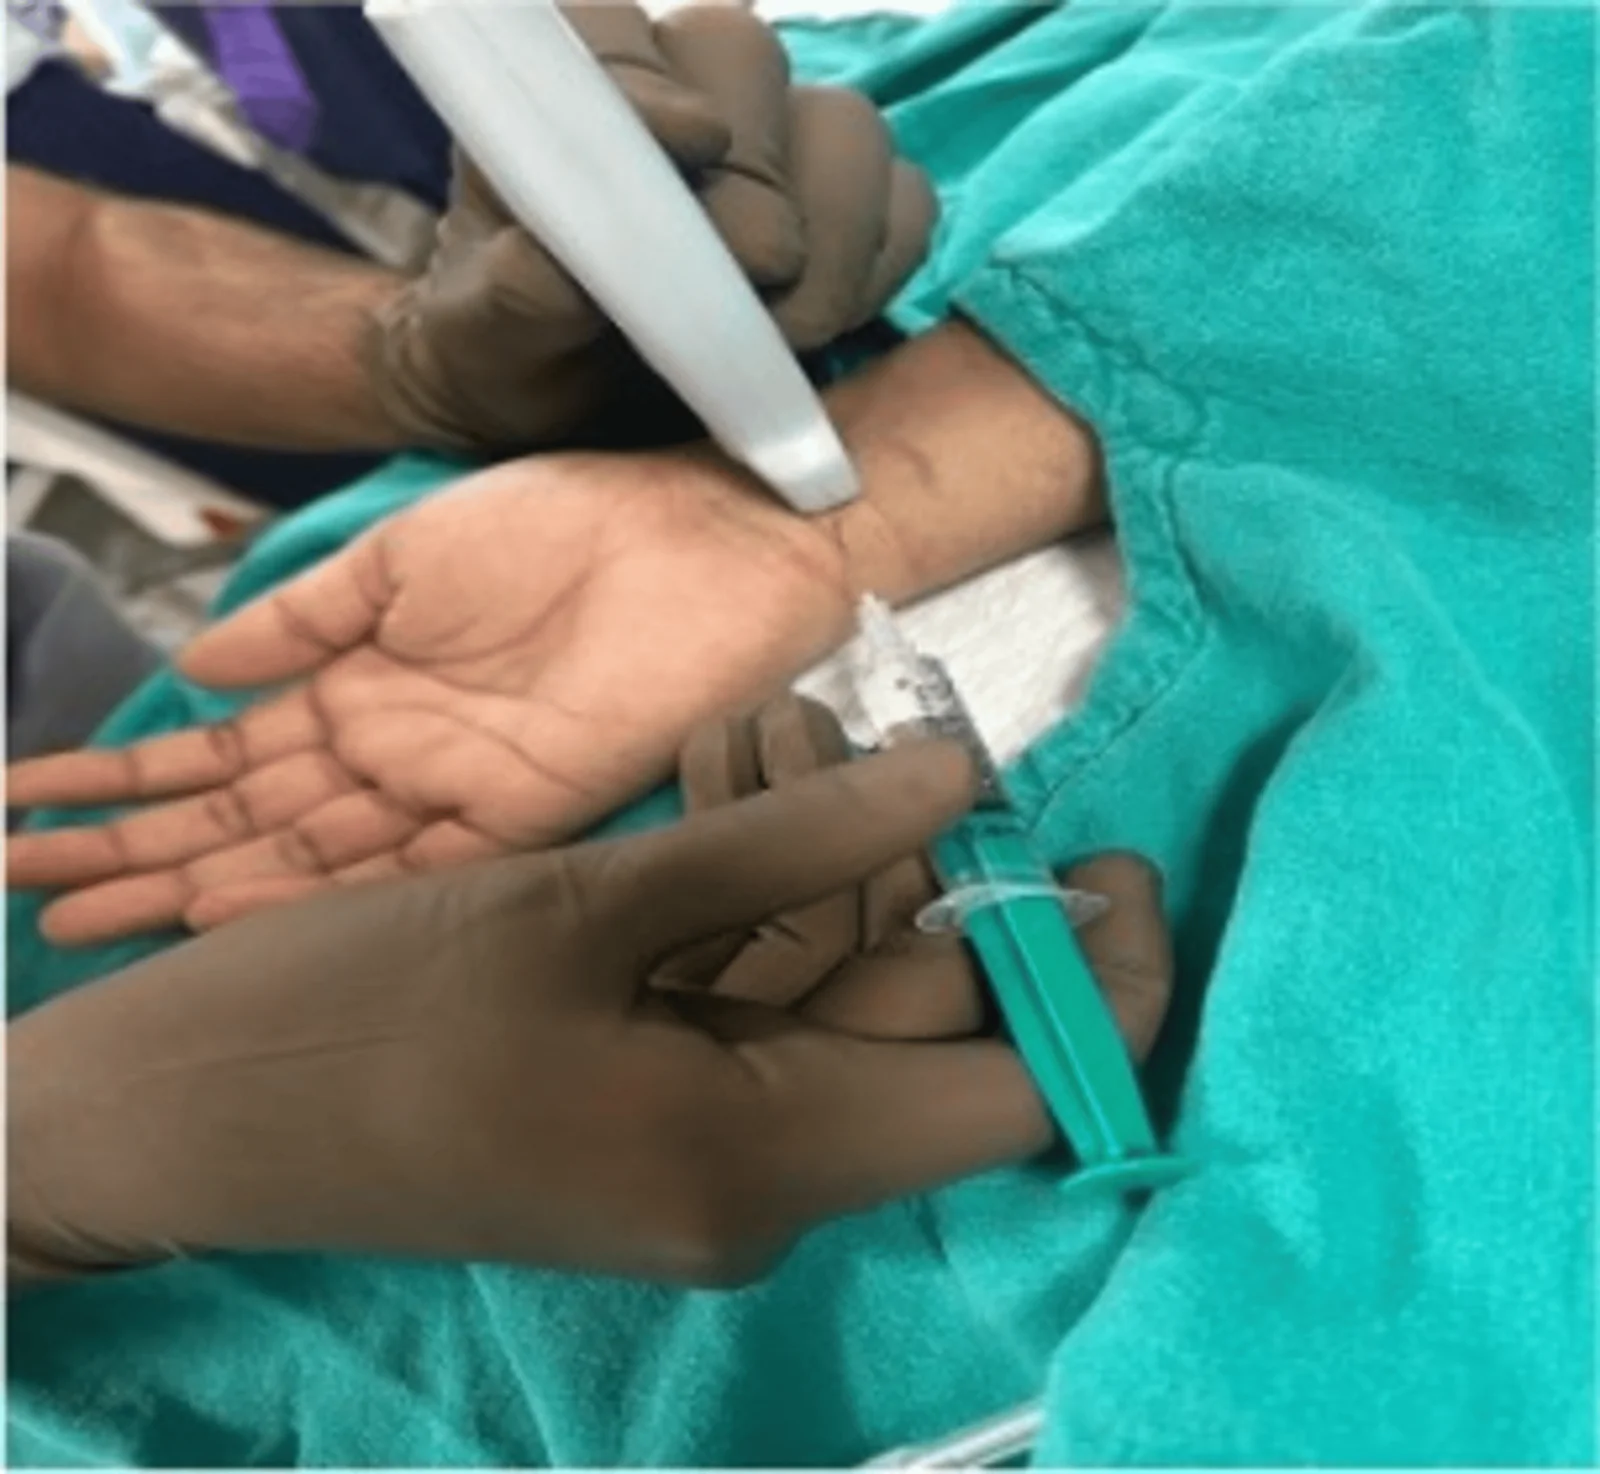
Ultrasound-guided in-plane needle placement after identification of the ulnar artery using color Doppler.
